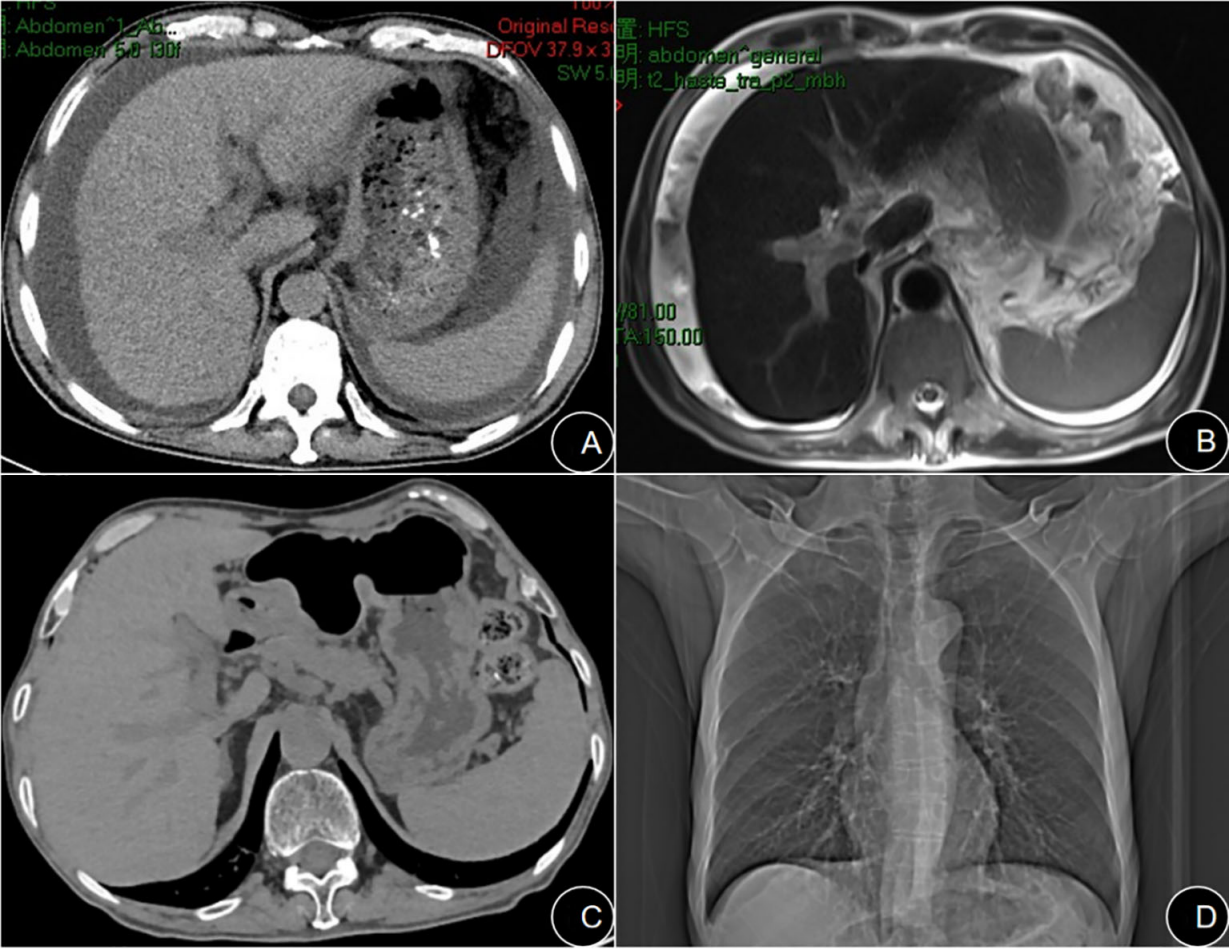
(A) Admission axial abdominal CT scan showing a widened portal vein diameter, massive ascites, and splenomegaly. (B) Admission T2-weighted abdominal MRI confirming ascites and heterogeneous liver parenchyma. (C) Follow-up axial abdominal CT scan after therapy demonstrates complete resolution of ascites and reduced splenic size. (D) Follow-up chest CT (bone window) reveals no osteolytic lesions.
